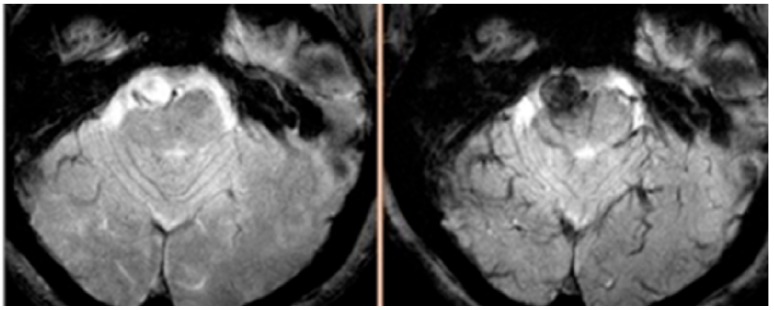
MRI, T2* GE sequence, from a patient showing early uptake of ferumoxytol. The aneurysm ruptured within three months.
